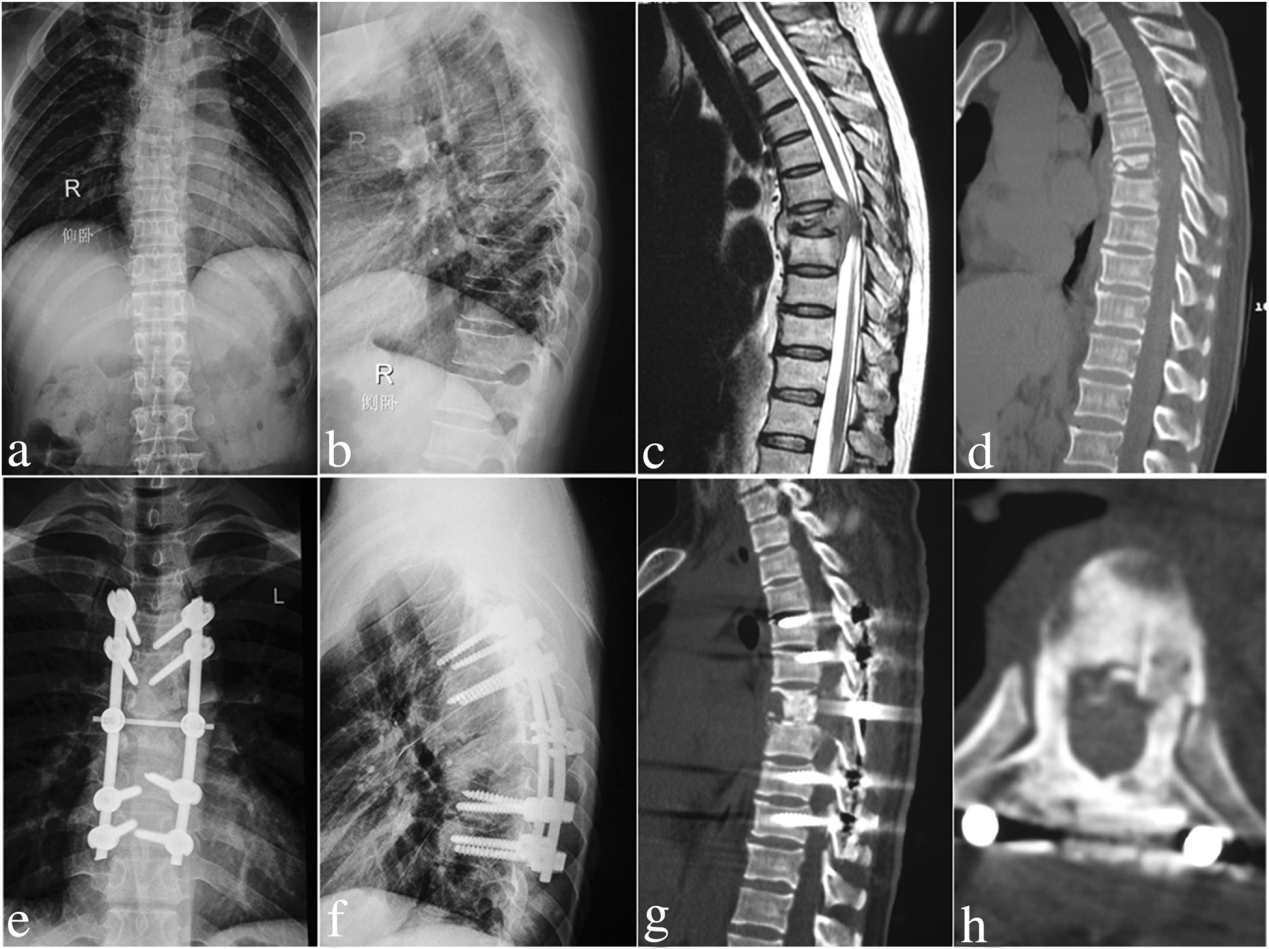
A 52-year-old female with T5/6 lesions was performed by posterior-only approach. a-d The pre-operative imaging data showed T5/6 vertebral bodies’ destructions with mild kyphosis deformity and spinal cord severely compressed. The postoperative anterior-posterior (e) and lateral X-ray (f) indicated that the kyphosis got obviously improved by posterior long-segment fixation. Sagittal and coronal CT-scan (g, h) showed satisfied allograft fusion without relapse of Pott’s disease at the 9 months of post-operation
